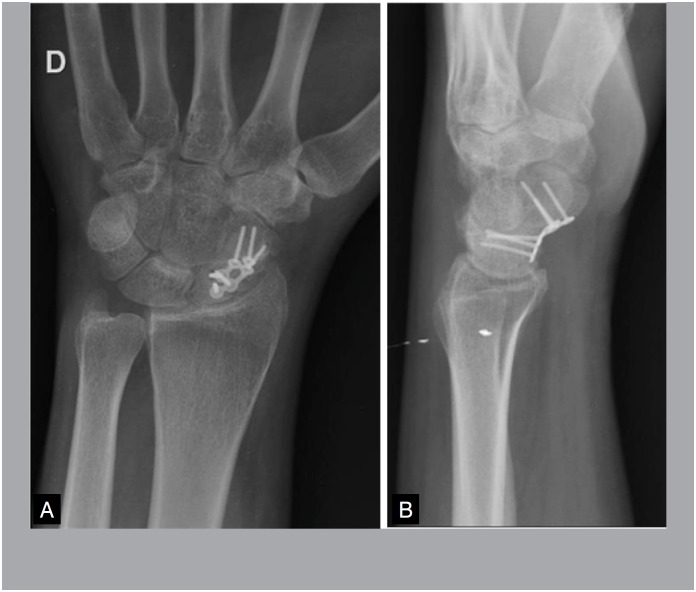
Postoperative X-ray of pseudoarthrosis fixation.
